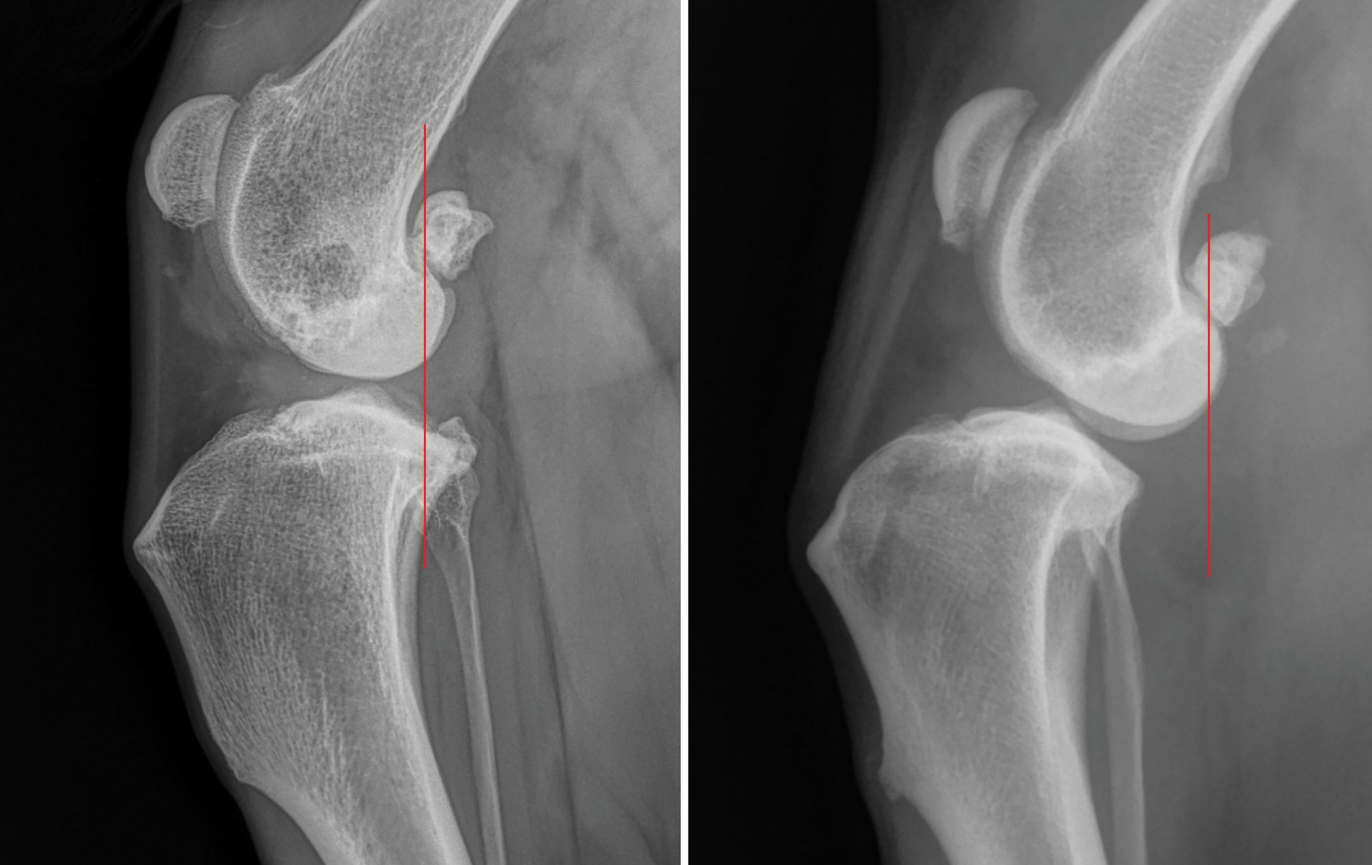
Examples of unstressed, standing angle, mediolateral radiographs of the stifle without (A) and with (B) cranial tibial translation.
